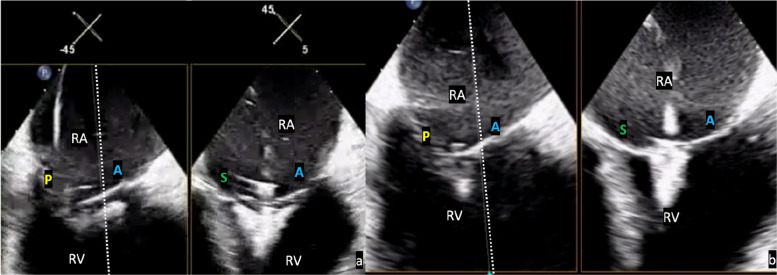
The 3D intracardiac echocardiography imaging can serve as important tool when the transesophageal echocardiography imaging is suboptimal to guide tricuspid valve (TV) leaflet grasping. The use of –45°/+ 45° view together with a left flexion could help to get the adequate images for anteroseptal leaflet grasping in this situation. A TV leaflet capture by TriClip. B Adequate TV leaflet capture. A, anterior leaflet; P, posterior leaflet; RA, right atrium; RV, right ventricle; S, septal leaflet
